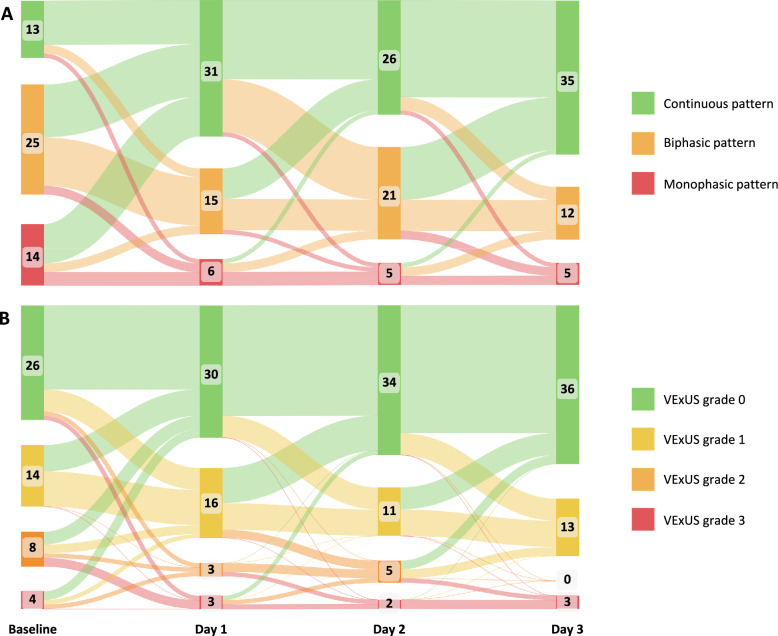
Temporal changes in intrarenal venous flow patterns and VExUS grades over the first three days. A Intrarenal venous flow patterns; B VExUS grades. VExUS, Venous Excess Ultrasound
